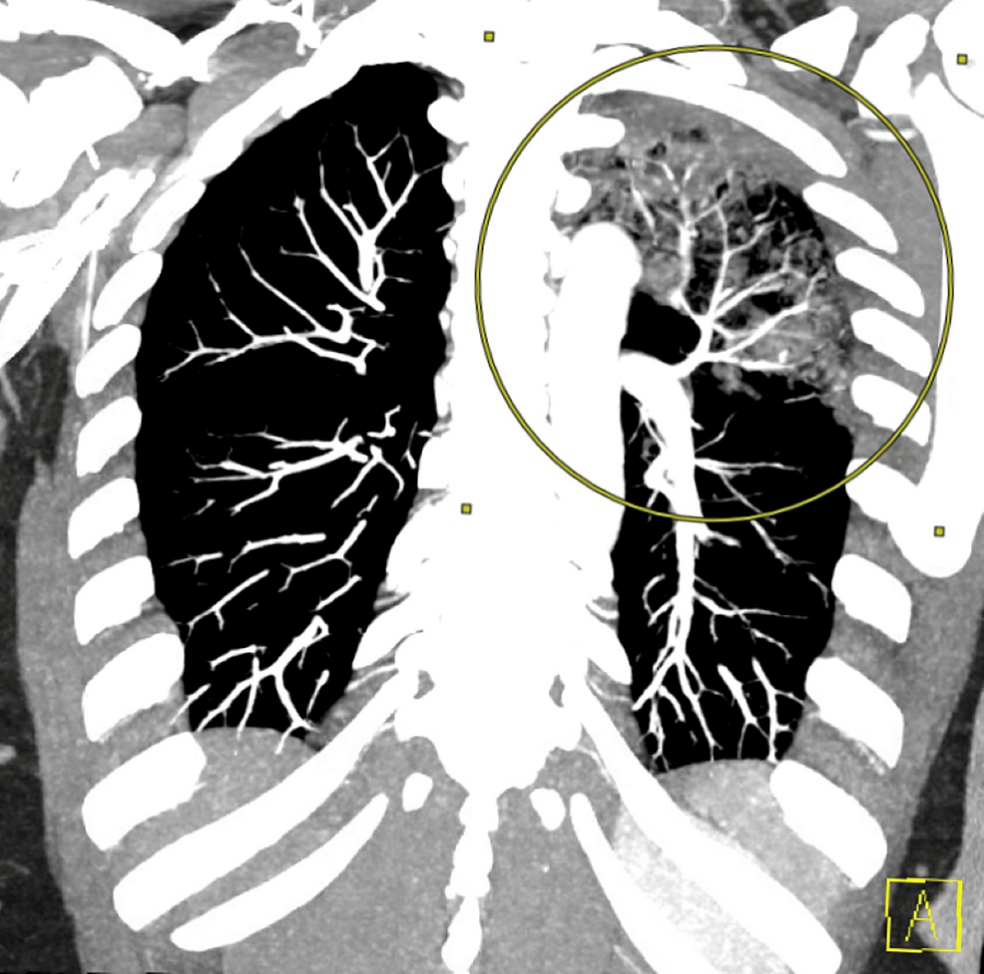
Chest CT with angiography revealing a large left upper lobe pneumonia (yellow circle)
